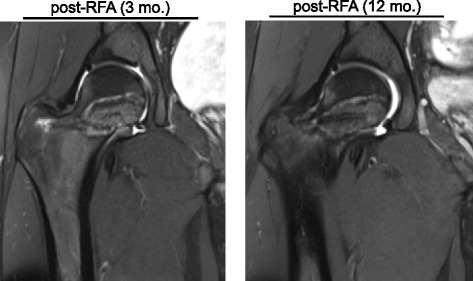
Femoral neck osteonecrosis after RFA. Proton density (PD)-weighted fat-suppressed (FS) turbo spin echo (TSE) MRI images 3 and 12 months after the intervention. There is a hyperintense signal indicating an excessive necrosis zone around the insertion channel of the ablation probe
